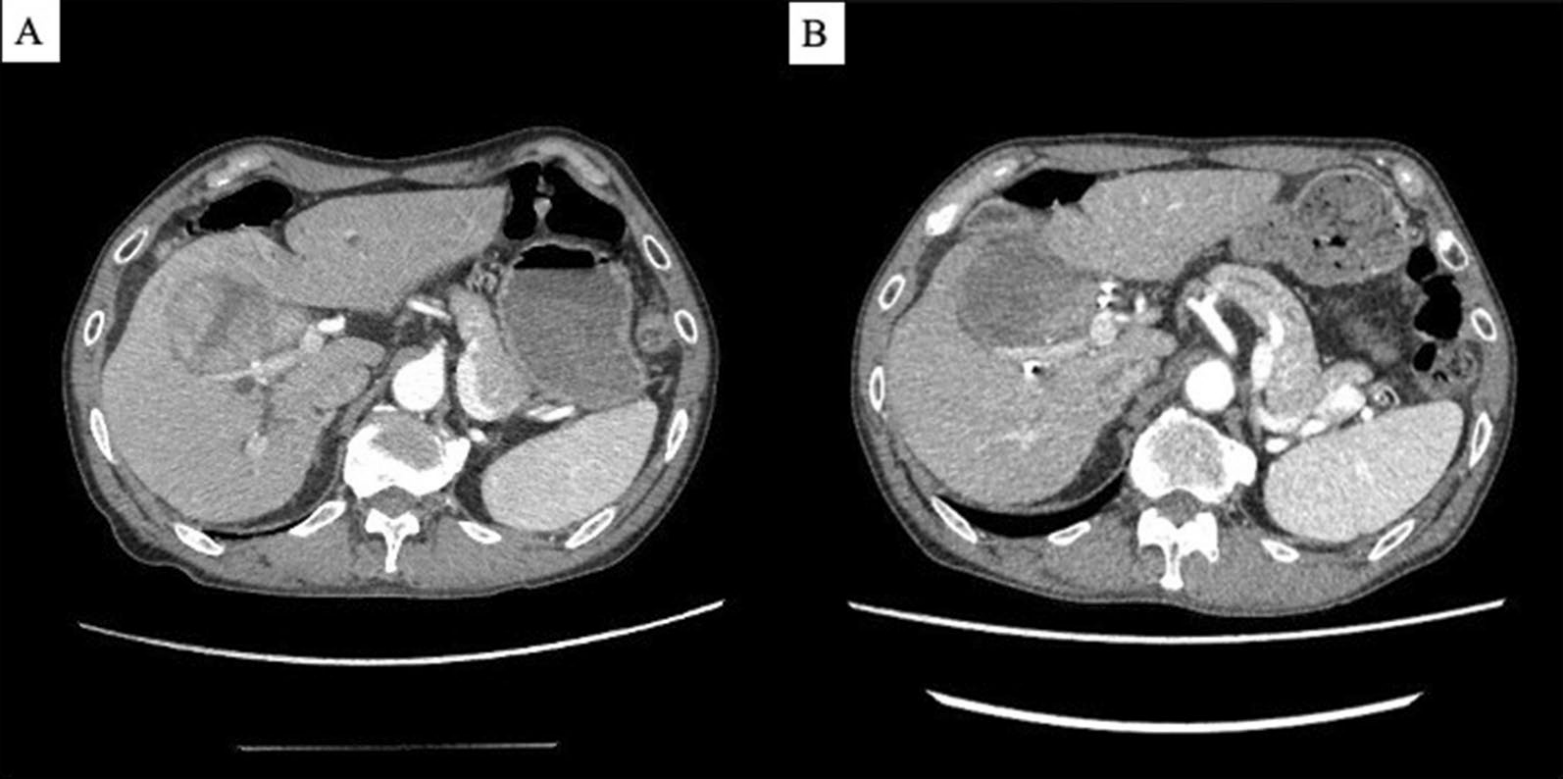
Preoperative contrast-enhanced computed tomography (CT) of the arterial phase. Huge hepatocellular carcinoma located in the hepatic hilum, before (A) and after (B) atezolizumab and bevacizumab treatment. The tumor viability seemed reduced; note that the CT attenuation number is diffusely decreased in B
